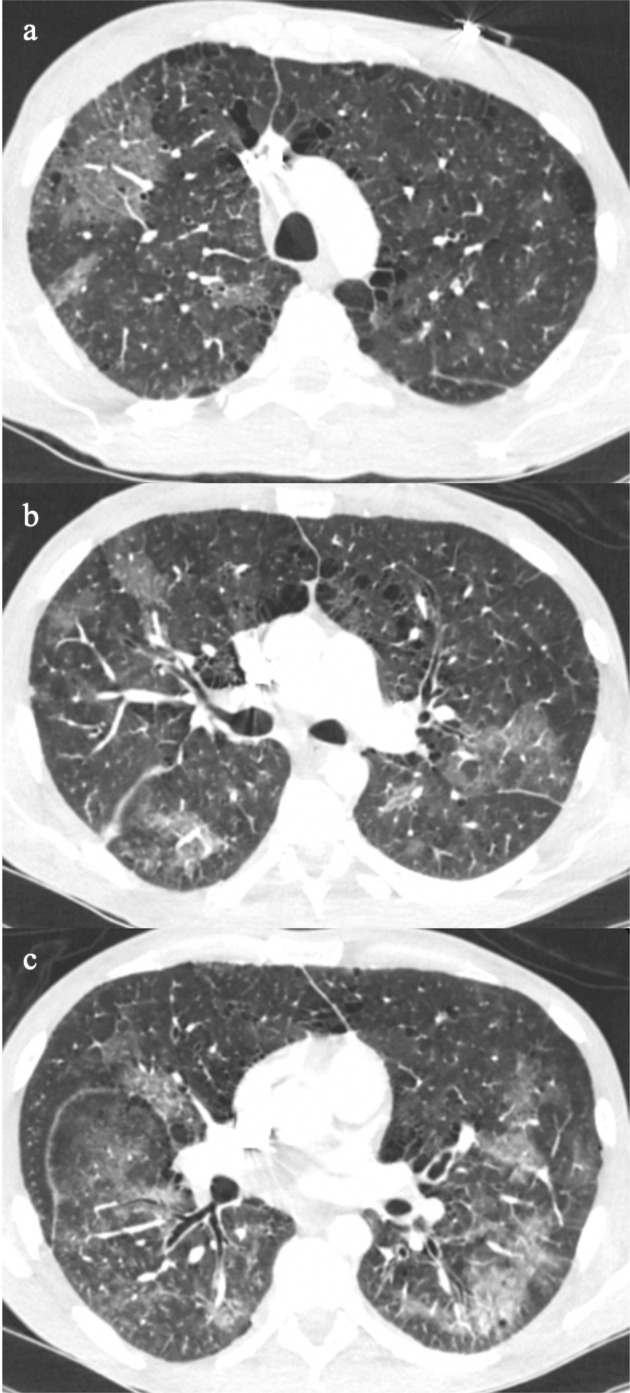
Patient was newly diagnosed with HIV and presented with fever and cough. The chest CT showed bilateral patchy ground glass opacification and slightly worse in the lower lobes (9a–c). The pattern is non-specific and could be due to various opportunistic organisms. Later, he was confirmed to have Epstein-Barr viral infection, which induced HLH. HLH, haemophagocytic lymphohistiocytosis.
